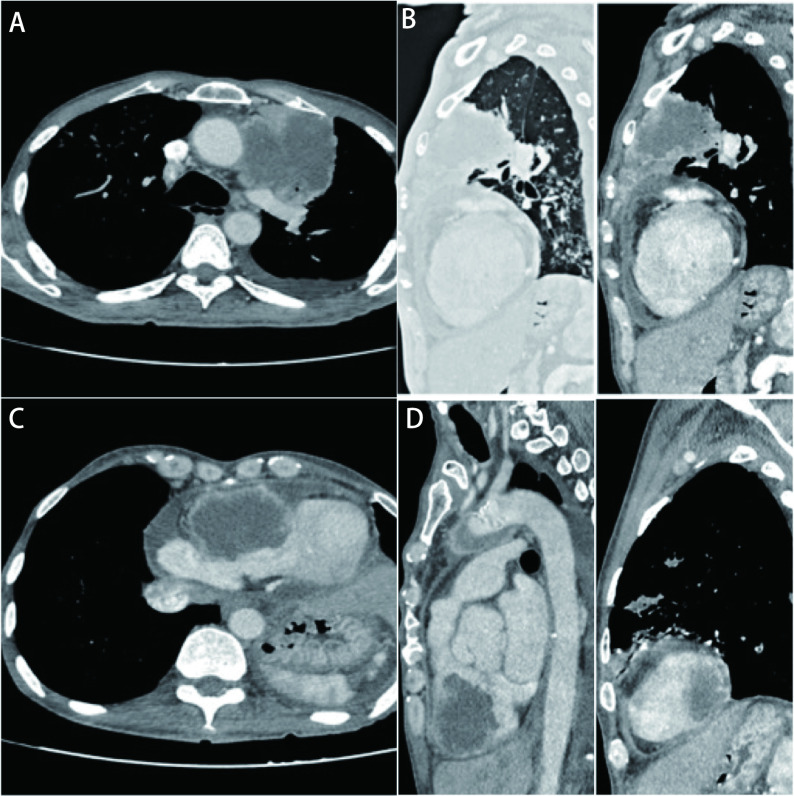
CT images. A: Axial cut showing a well-defined heterogeneously enhancing lesion with irregular margins noted in left upper lobe and bilateral multiple nodules and minimal pleural effusion on left side; B: Sagittal cut showing a well-defined heterogeneously enhancing lesion with irregular margins noted in left upper lobe; C: Axial cut showing a heterogeneous opacity in right ventricle of heart, exophytic and completely intraluminal with moderate pericardial effusion; D: Sagittal cut showing a heterogeneous opacity in right ventricle of heart, exophytic and completely intraluminal and a small heterogeneous opacity along lateral left ventricular wall. CT: computed tomography.
